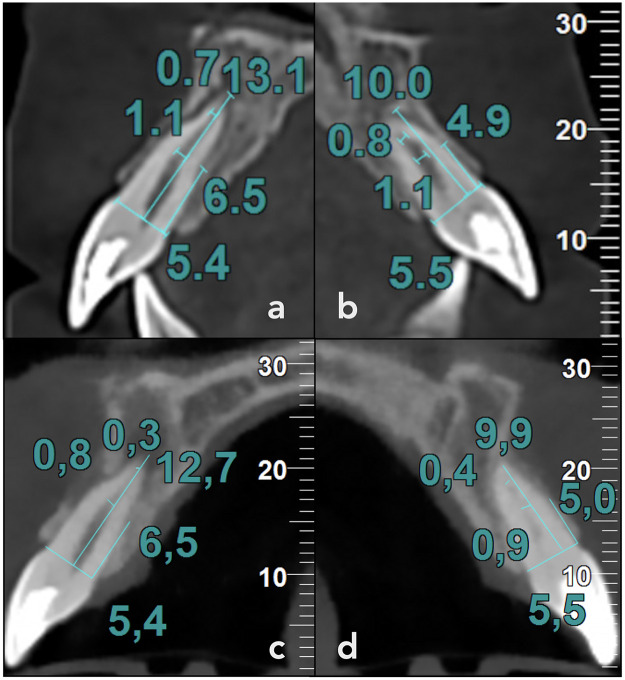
Comparison of CBCT images of PRF and BC-treated teeth. (a) Tooth #11 at 28-month (PRF). (b) Tooth #21 at 28-month (BC). (c) Tooth #11 at 80-month (PRF). (d) Tooth #21 at 80-month (BC).
